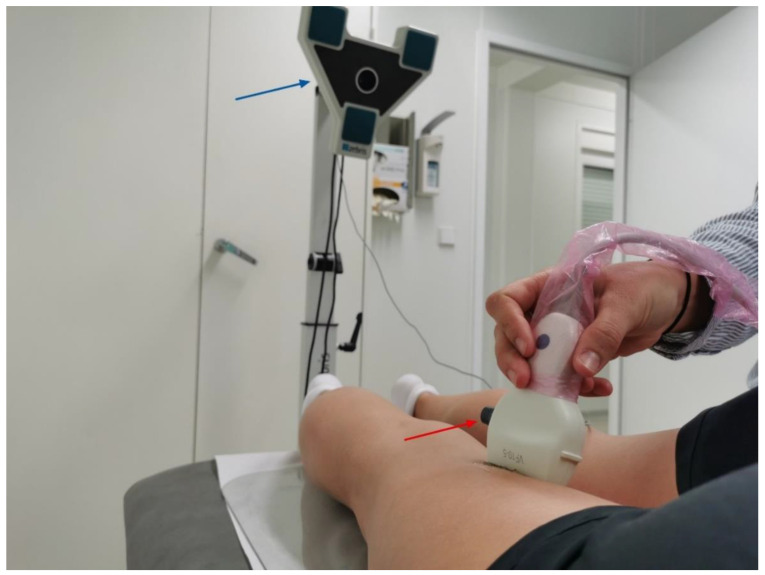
Ultrasound-based, three-dimensional movement analysis system. The photo shows the microphone of the device (blue arrow) and a marker (red arrow) attached to the transducer. The marker constantly sends an acoustical signal which is recorded by the microphone. As the transducer (and with this, the marker) is moved over the marked 3 cm distance on the skin, the travel time changes, which indicates probe (marker) movement.
